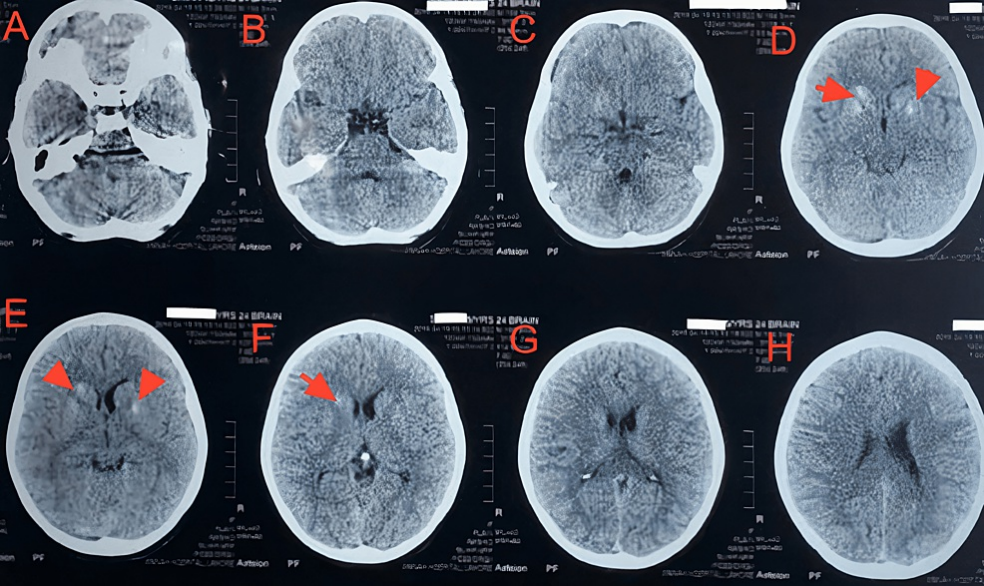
Plain CT brain, axial sections The red arrows in sections D, E, and F depict basal ganglia calcifications, which constitute Fahr's syndrome, present in patients with hypoparathyroidism and APS-1 APS-1: Autoimmune Polyglandular Syndrome type 1
